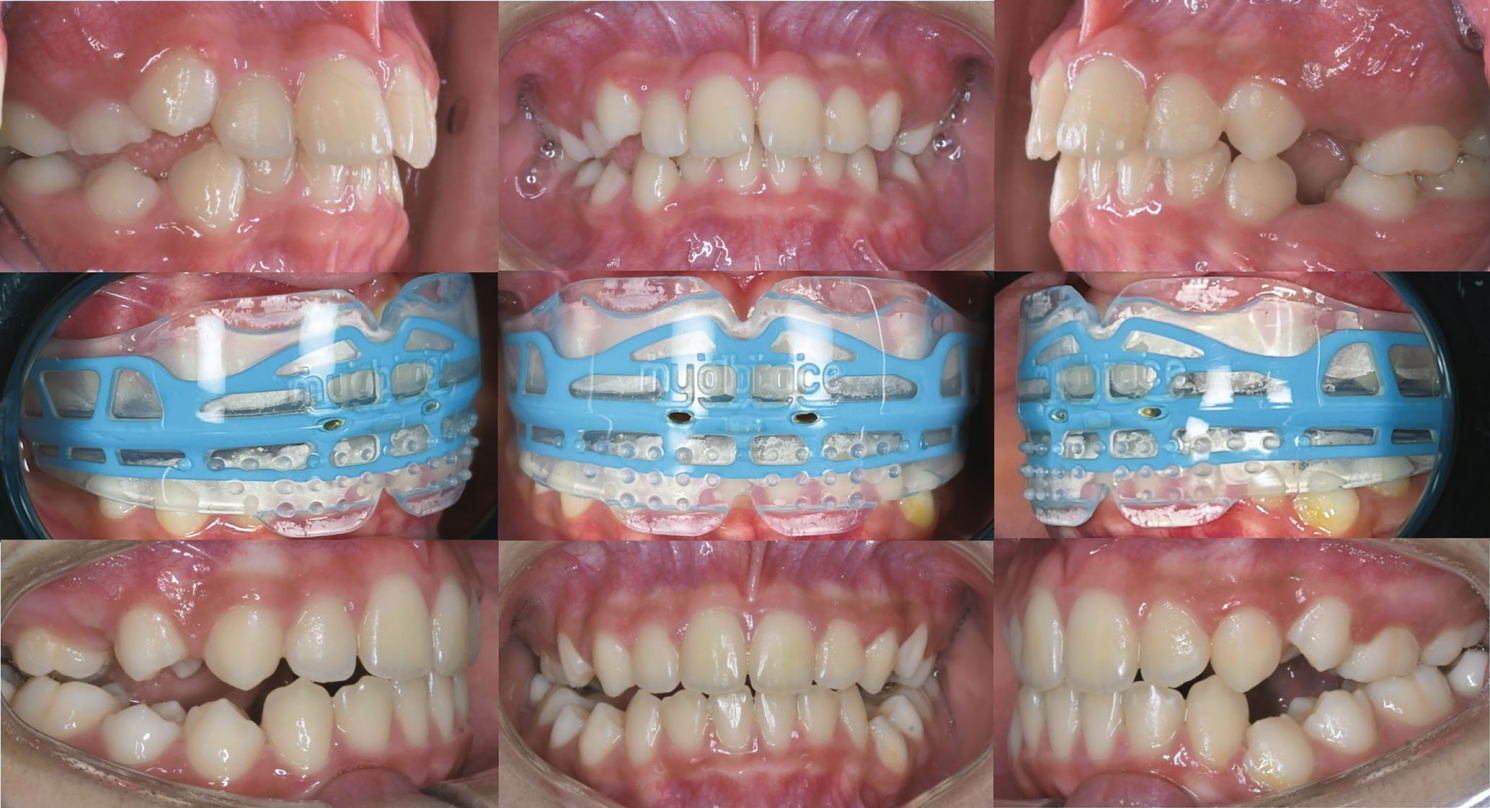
Pre- and post-treatment intraoral radiographs, before treatment (upper), during treatment (middle), and after treatment (lower).
